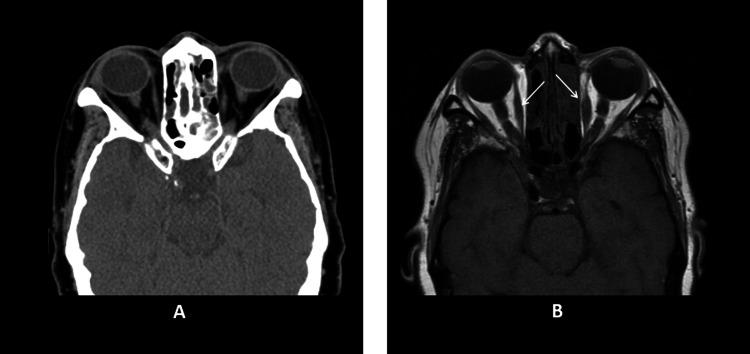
Serial imaging CT and MRI with contrast. Follow-up axial CT image and T2-weighted MR image showing interval resolution of the previously seen hematoma in the left medial rectus muscle with residual fatty stranding, abnormal shape of the muscle, and thinned tendon with irregular margins (A, B). Compare the abnormal left to the normal right (straight arrows) (B).
